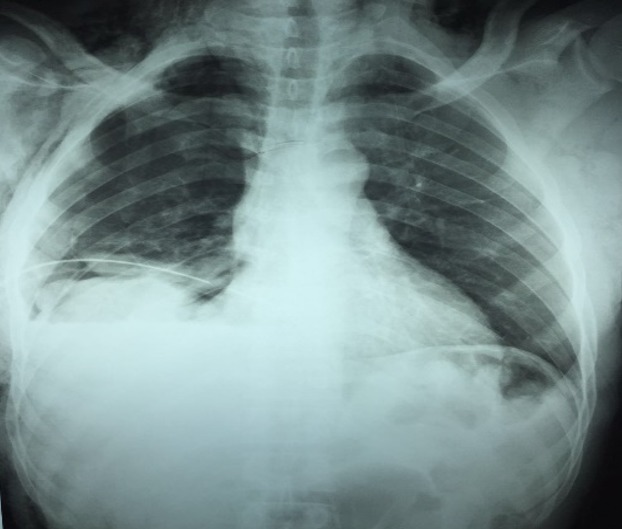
Preoperative erect chest x-ray of case 1 showing an irregularly shaped homogenous opacity in the right lower zone, loss of the right hemidiaphragmatic contour, a chest tube and minimal subcutaneous emphysema
